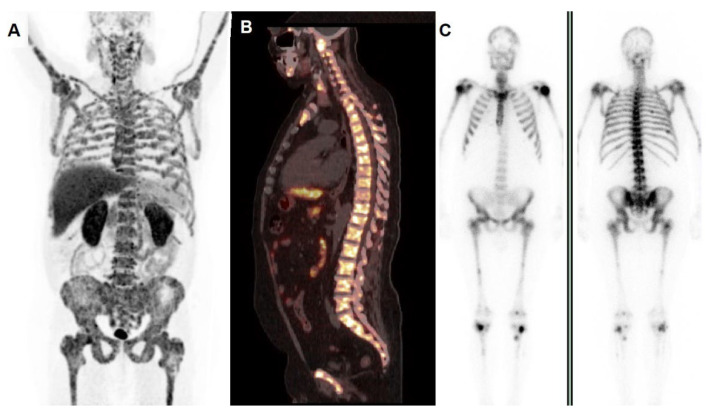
A 57-year-old man, diagnosed with locally advanced PCa in 2019 (Gleason score 4 + 5, ISUP 5), progressive after a sequence of various therapeutic regimens due to skeletal metastases (ADT, second generation androgens, taxane chemotherapy), positive for ATM germline mutation at NGS analysis. He was referred to our facility for ^223^Ra-therapy: pre-therapy whole body PET/CT with ^18^F-choline (A) showed increased tracer incorporation in the entire skeleton, as well depicted also by sagittal fused PET/CT of the spine (B). PET/CT’s findings were consistent with bone scan (C), left side—anterior view, right side—posterior view) demonstrating a typical pattern of “super bone”. After 2 cycles, a biochemical ALP response was registered (79 U/L post 2 cycles vs. 326 U/L at baseline). Unfortunately, the patient discontinued therapy due overlapping pulmonary complications.
